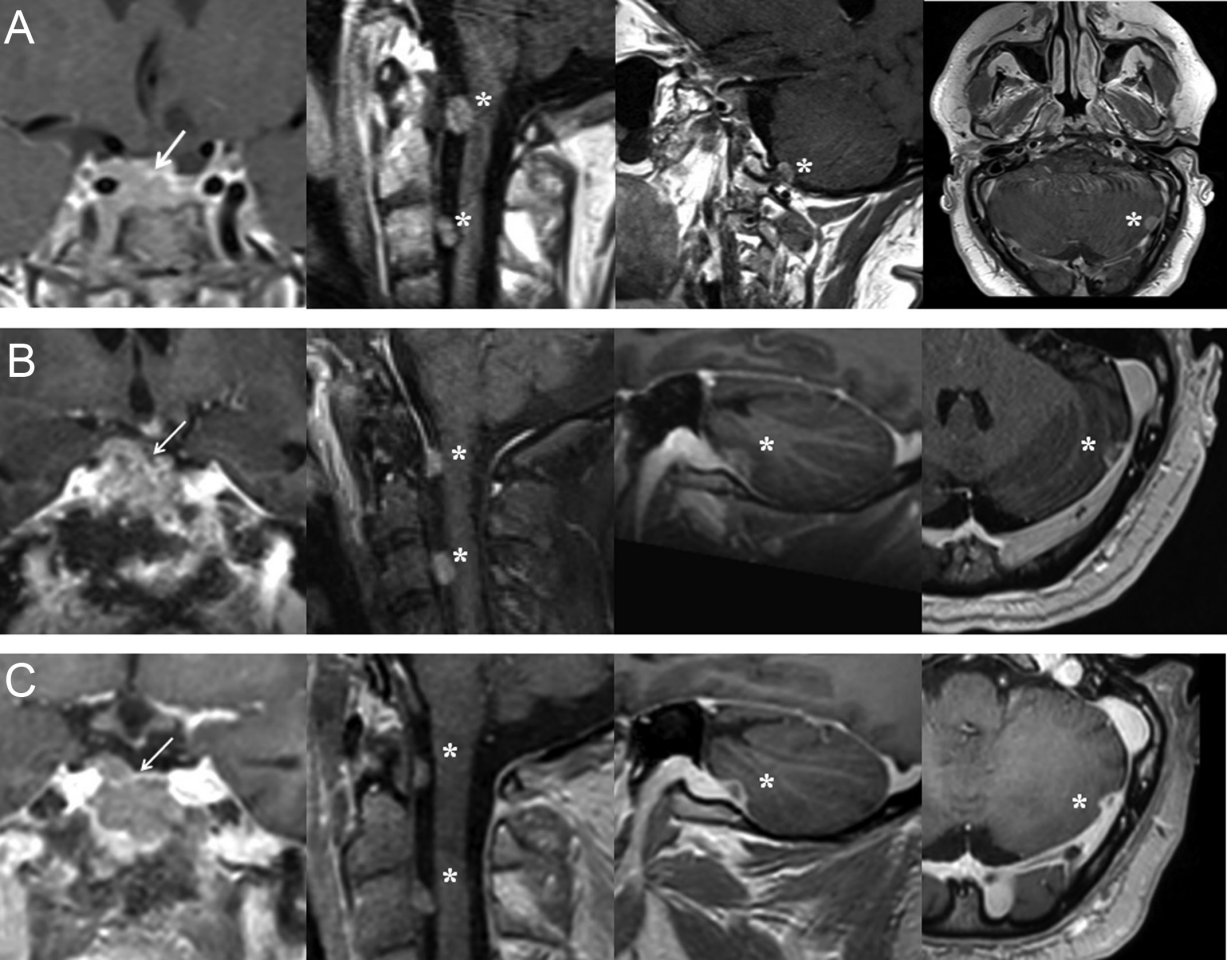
Gadolinium-enhanced T1-weighted magnetic resonance imaging of the pituitary carcinoma. Panel A shows invasion of the cavernous sinus (arrow) and the metastases of the posterior fossa, left cerebellum and cervical drop metastases at the level of the dens and the third cervical vertebra (asterix) (May 2018). Panel B shows evaluation of the pituitary carcinoma after nine cycles of TMZ treatment (April 2019). Panel C shows stable disease (irRECIST criteria) 1 year after initiation of ICI (April 2020). IrRECIST, immune-related Response Evaluation Criteria in Solid Tumors; ICI, Immune Checkpoint Inhibitors.
